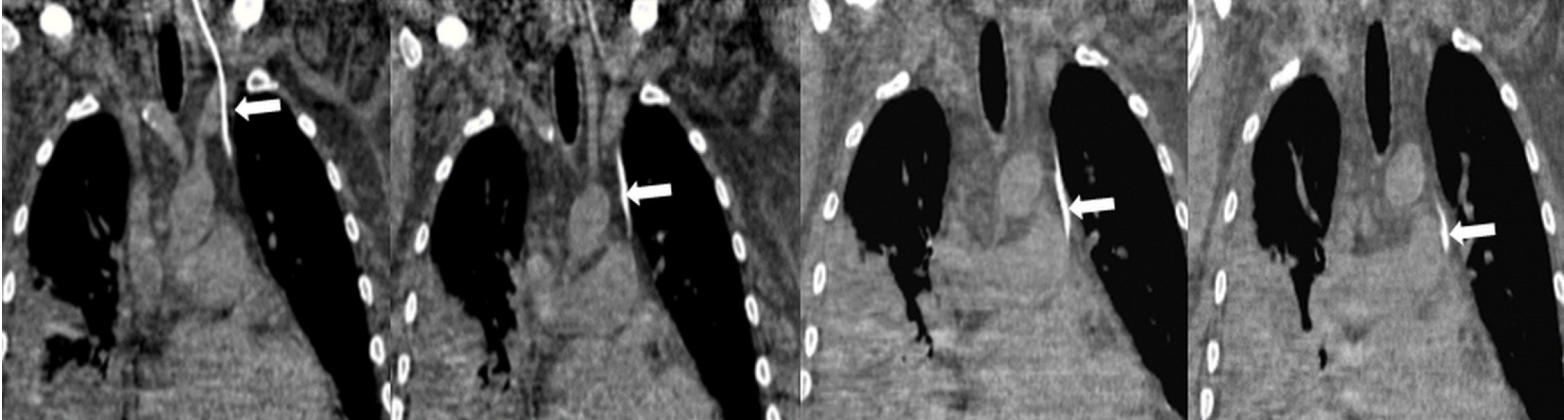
Chest computed tomography without contrast (coronal sections) shows the course of the CVC (arrows) descending via the left internal jugular vein, crossing the left brachiocephalic vein, and then descending through the left pericardiophrenic vein. *CVC*, central venous catheter
